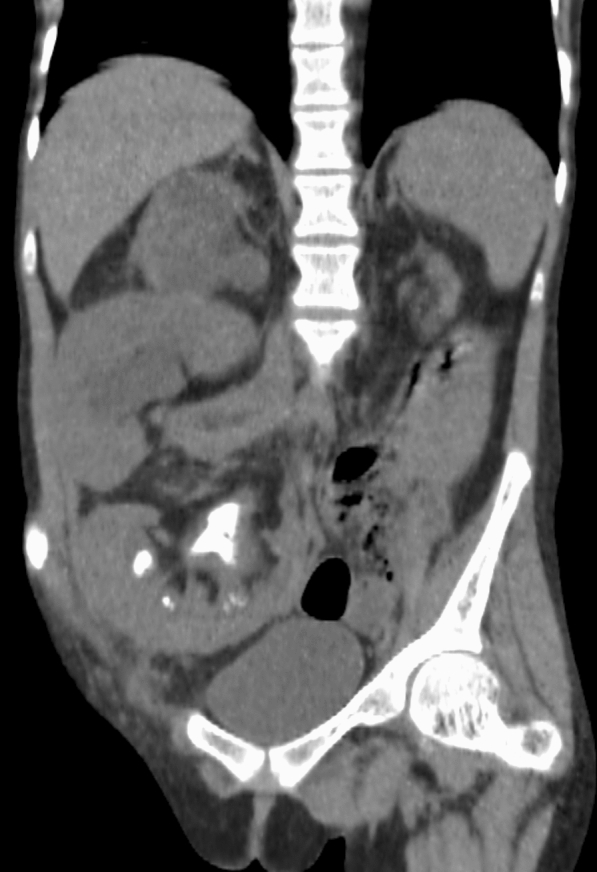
Preoperative CT scan shows incomplete staghorn calculi in right iliac fossa transplanted kidney and right native and flank transplanted kidneys dilated by ureteral stone
